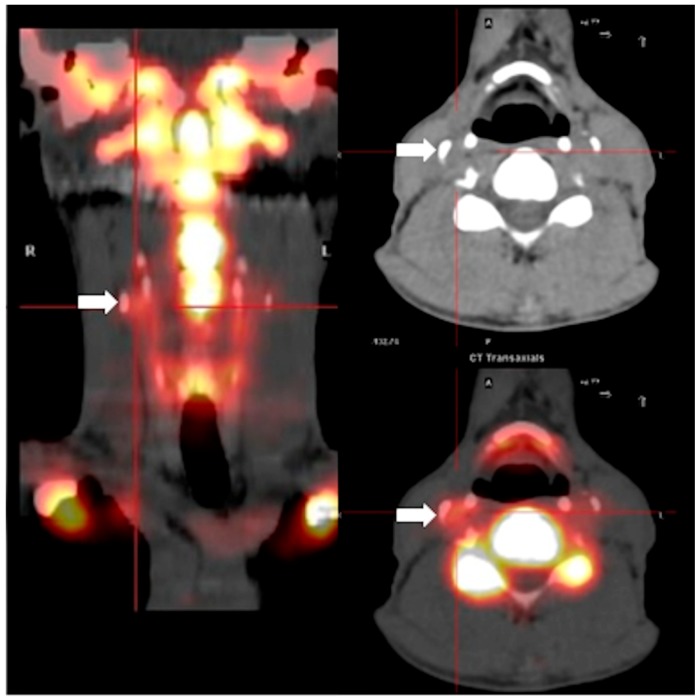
Example of carotid artery ^18^F-Sodium Fluoride uptake in a 50 years old patient with HIV. The image on the left is a coronal reconstrution of the PET image of the neck and base of the skull of this patient. The white arrow points at one of several areas of enhanced ^18^F-Sodium Fluoride uptake along the course of the right internal carotid artery. The image on the top right is an axial computed tomography section of the neck of the same patient, and the white arrow points at a calcified lesion corresponding to the lesion seen on the PET image on the left. The bottom right image is a fusion of axial PET and CT images; the white arrow points again at the same lesion shown in the axial CT image above, demonstrating ^18^F-Sodium Fluoride uptake in correspondence with the calcification.
